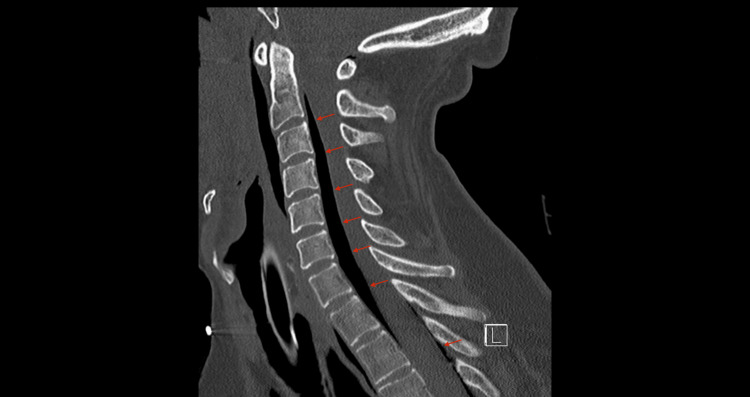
Sagittal CT reconstruction showing extensive cervicothoracic pneumorachis.
